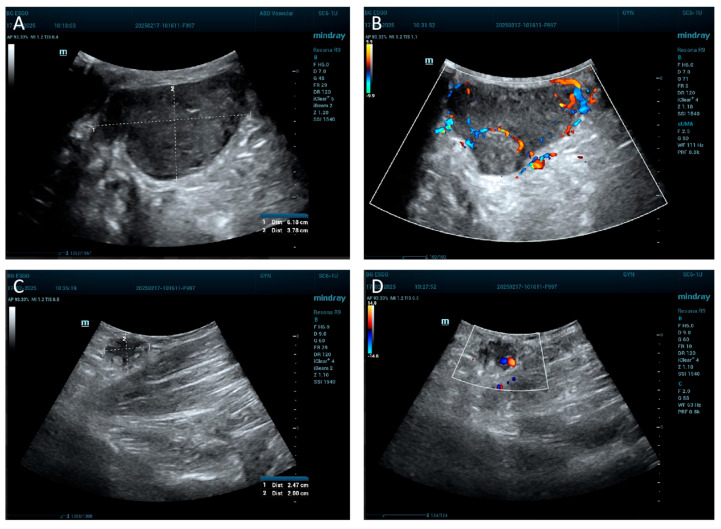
Ultrasound of the tumor formation and lymph node. (A) Ultrasound of the tumor formation. (B) Doppler ultrasound of the tumor formation. (C) Ultrasound of the lymph node. (D) Doppler ultrasound of the lymph node.
